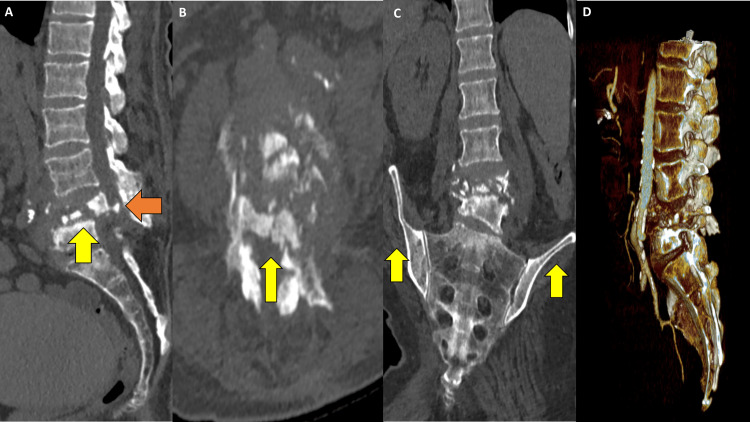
A) Sagittal multiplanar reconstruction of lumbar CT demonstrating a two-column L4 burst fracture with retropulsed fracture fragments traverse the complete anterior-posterior (AP) spinal canal (orange arrow). There are changes of bone resorption with two-column fracture of also the L5 superior endplate (yellow arrow); B) Axial view at the L4-5 level showing severe canal compromise (yellow arrow); C) Coronal view showing the right pelvic and lumbar tilt (yellow arrows) due to the burst fracture; D) Volume-rendered 3D reconstruction with sagittal clip plane through the left lateral vertebral body plane.
